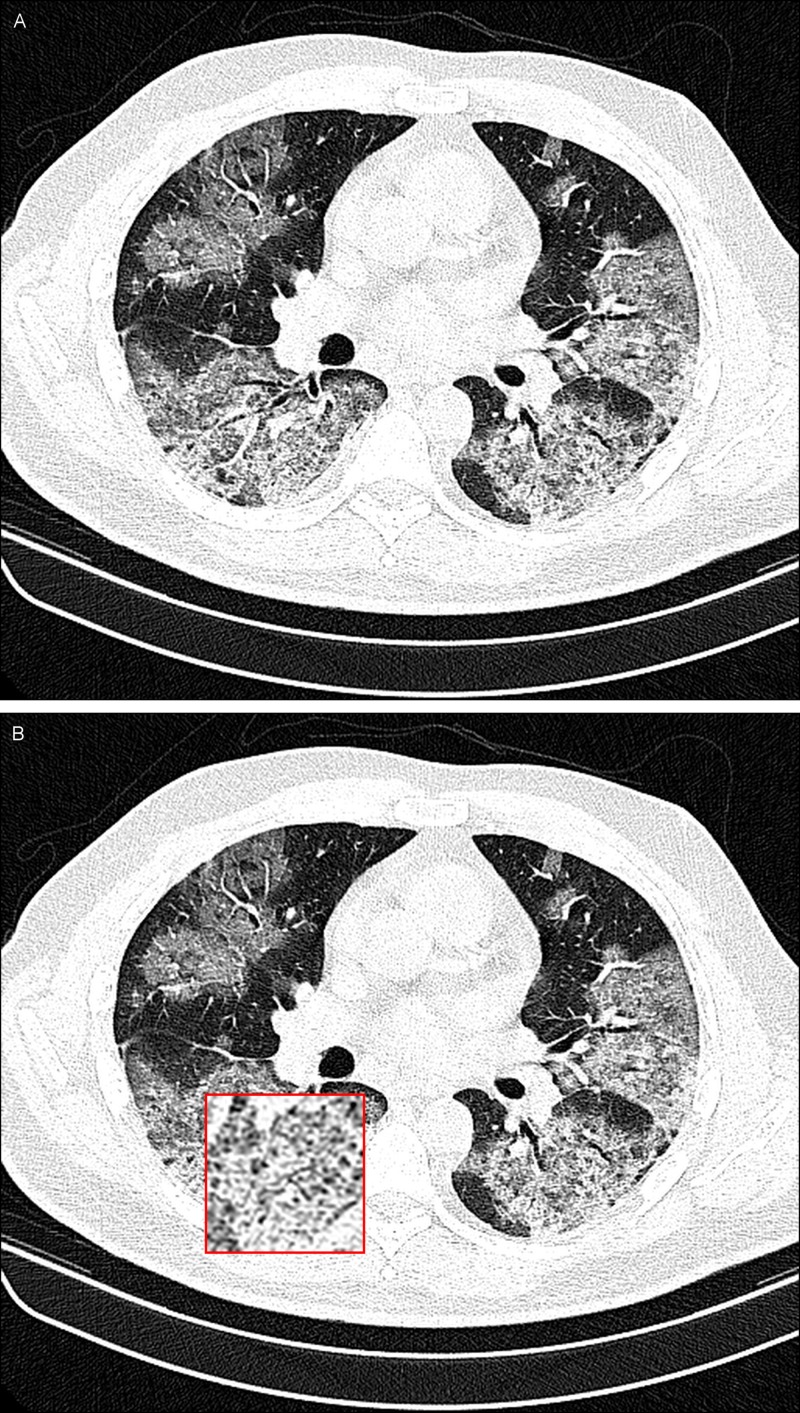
A 51-year-old man with COVID-19 infection. A noncontrast-enhanced CT image of the lungs demonstrating bilateral crazy paving in the posterior lobe of both lungs (A) and an inset magnified view of the lesion for better delineation at the location of crazy paving in the right lower lobe of the lung (B).
